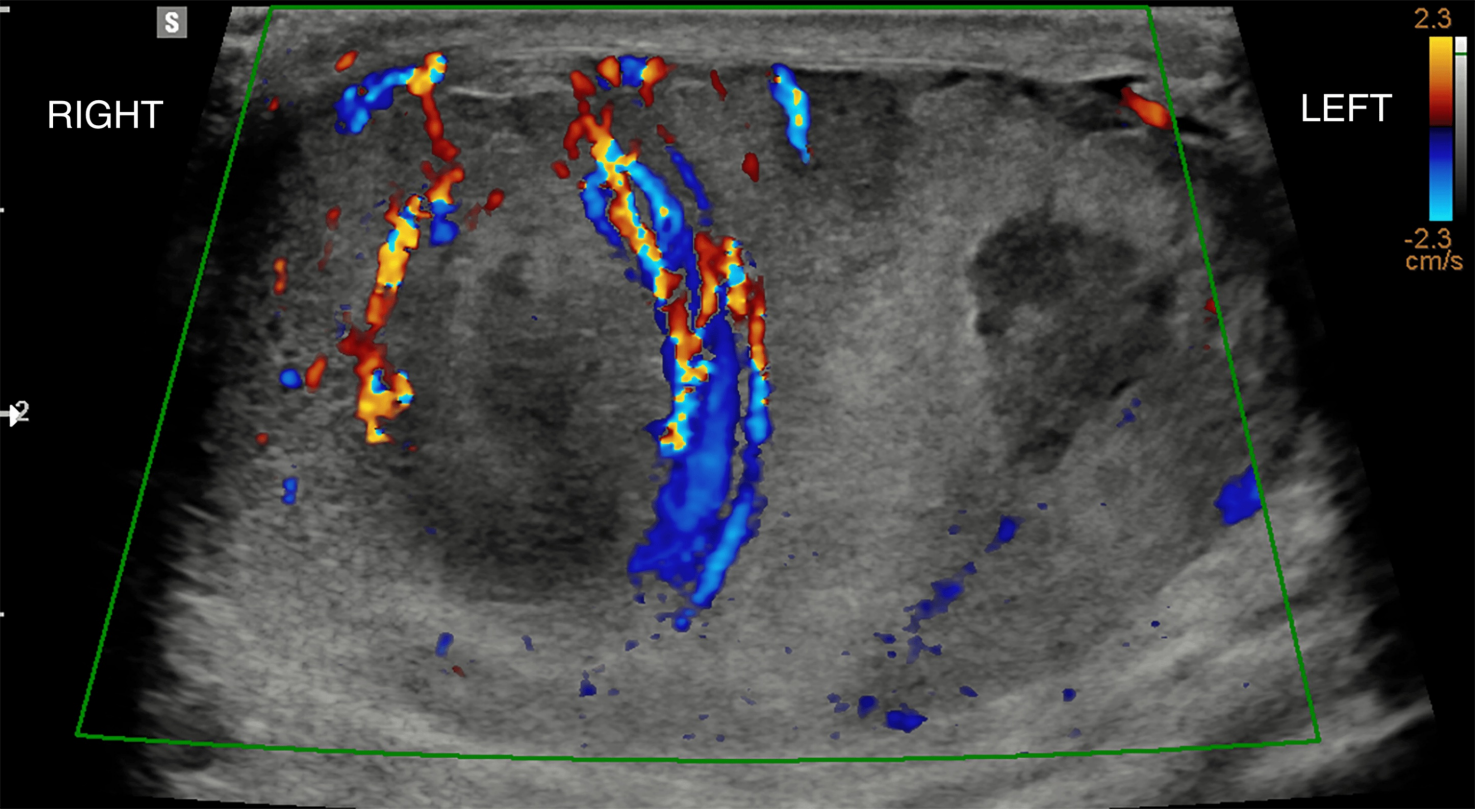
Scrotal ultrasound demonstrating hyperaemia of the testis and epididymis bilaterally Significantly worse on the right testis compared to the left testis.
